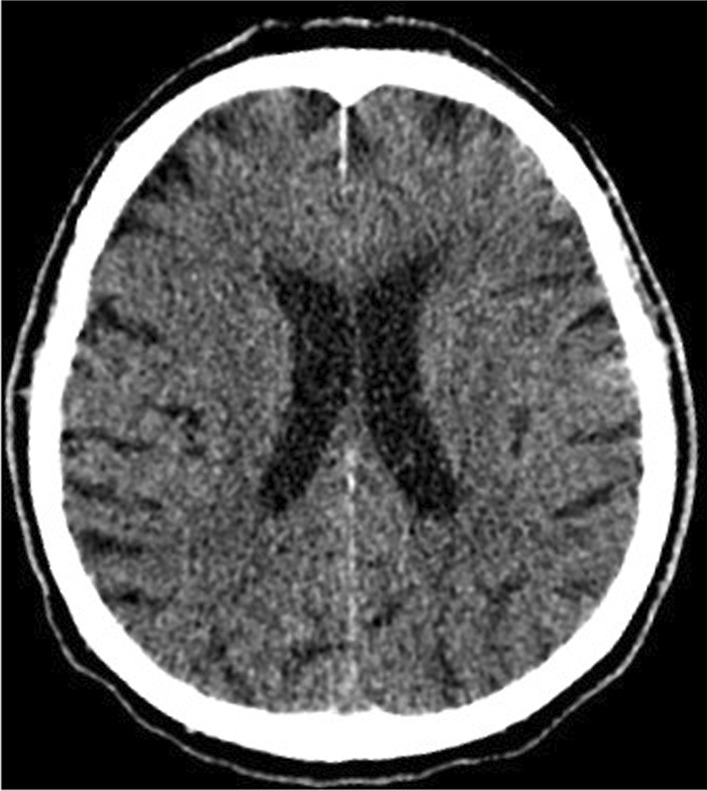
Brain computed tomography (axial scan): no evidence of intracranial hemorrhage. Features of leukoaraiosis. Ventricles of normal volume. Brain structures without displacement. Skull bones in the study area without traumatic injuries
